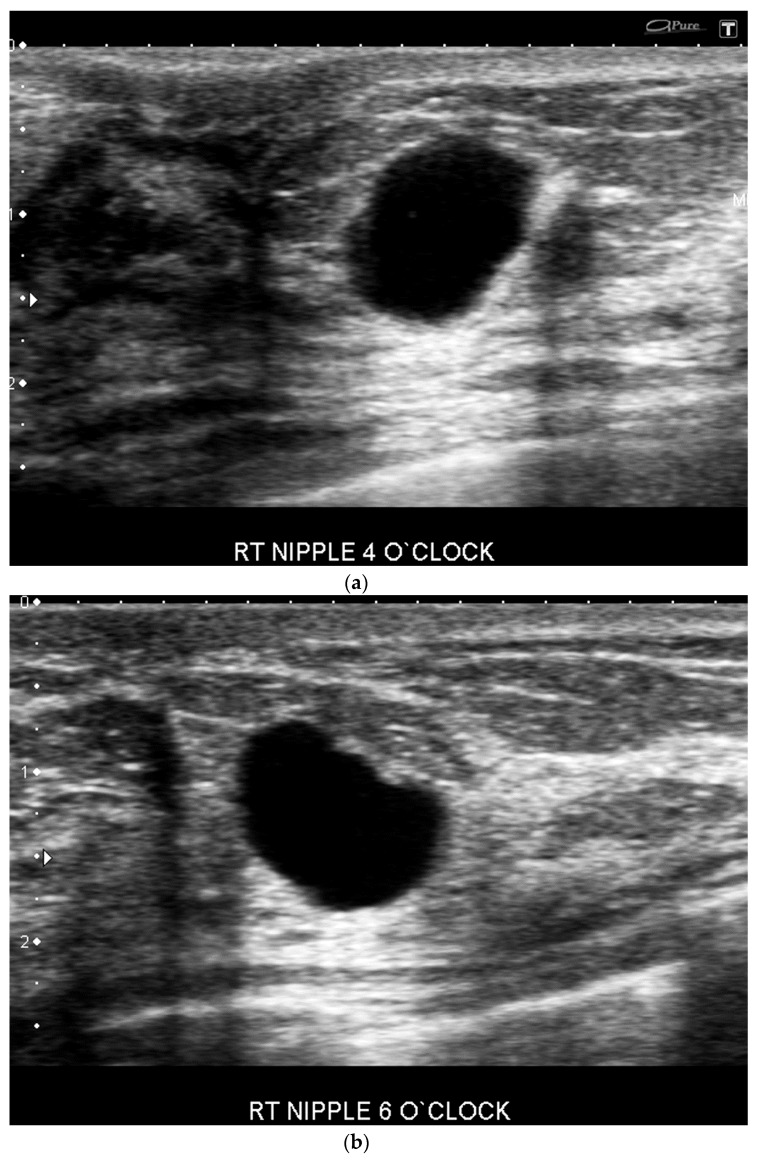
Different locations of a same retroareolar lesion depending on the breast imager. A retroareolar simple cyst is labeled sonographically at 4 o’clock by one breast imager (a) and 6 o’clock (b) by another one.
